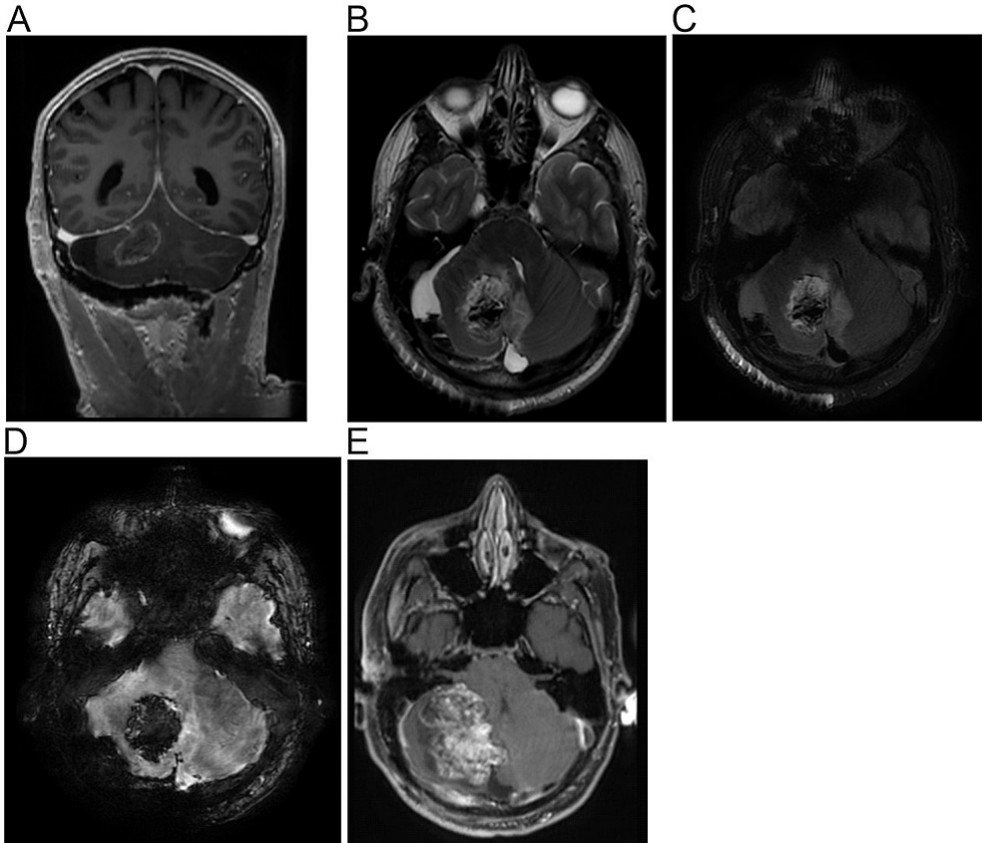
MRI of the brain one day and four weeks post craniotomy (A-D) MRI [(A): T1 (longitudinal relaxation time) without contrast, (B): T2 (transverse relaxation time), C: Fluid-attenuated inversion recovery (FLAIR), and D: Susceptibility-weighted angiography (SWAN)] of the brain post craniotomy with EVD. Postsurgical changes of right suboccipital craniotomy and resection of the right cerebellar tumor. Blood present within the resection cavity and at the periphery limited evaluation for enhancement. However, there was no evidence of intra- or perilesional enhancement to suggest residual disease. (E) MRI of the brain with contrast four weeks post craniotomy. A mixed solid and cystic mass in the right cerebellar hemisphere with a heterogeneous enhancement of the solid component that measures approximately 63 x 52 x 34 mm engulfing the prior resection cavity. The lesion extends to the left cerebellar hemisphere and causes a mass effect on the right side of the brainstem, and results in effacement of the fourth ventricle. The mild dilatation of the lateral and third ventricles is suggestive of developing hydrocephalus.
